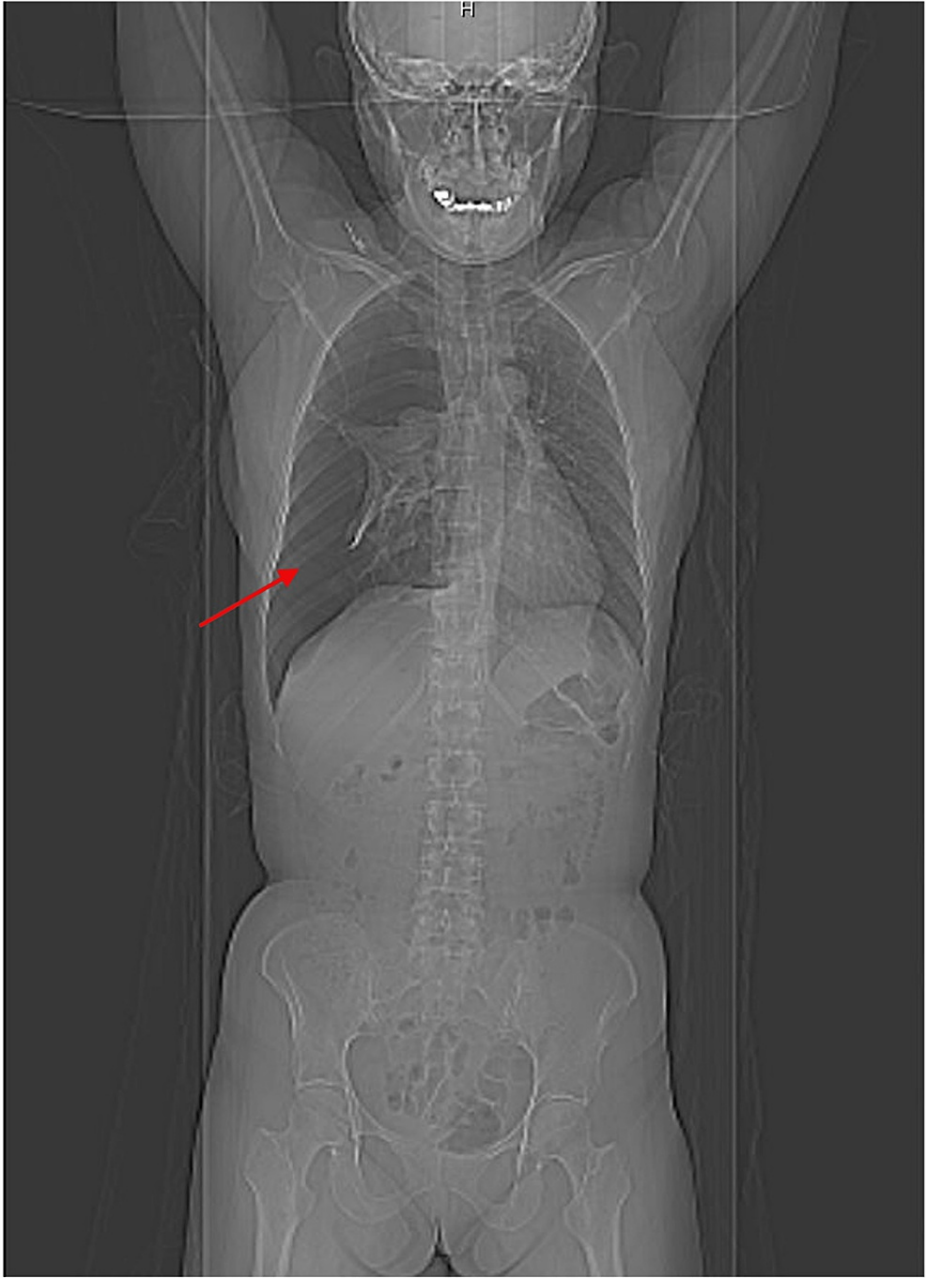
PET/CT showing hyperlucency of the right-sided lung (arrow) in coronal view.
